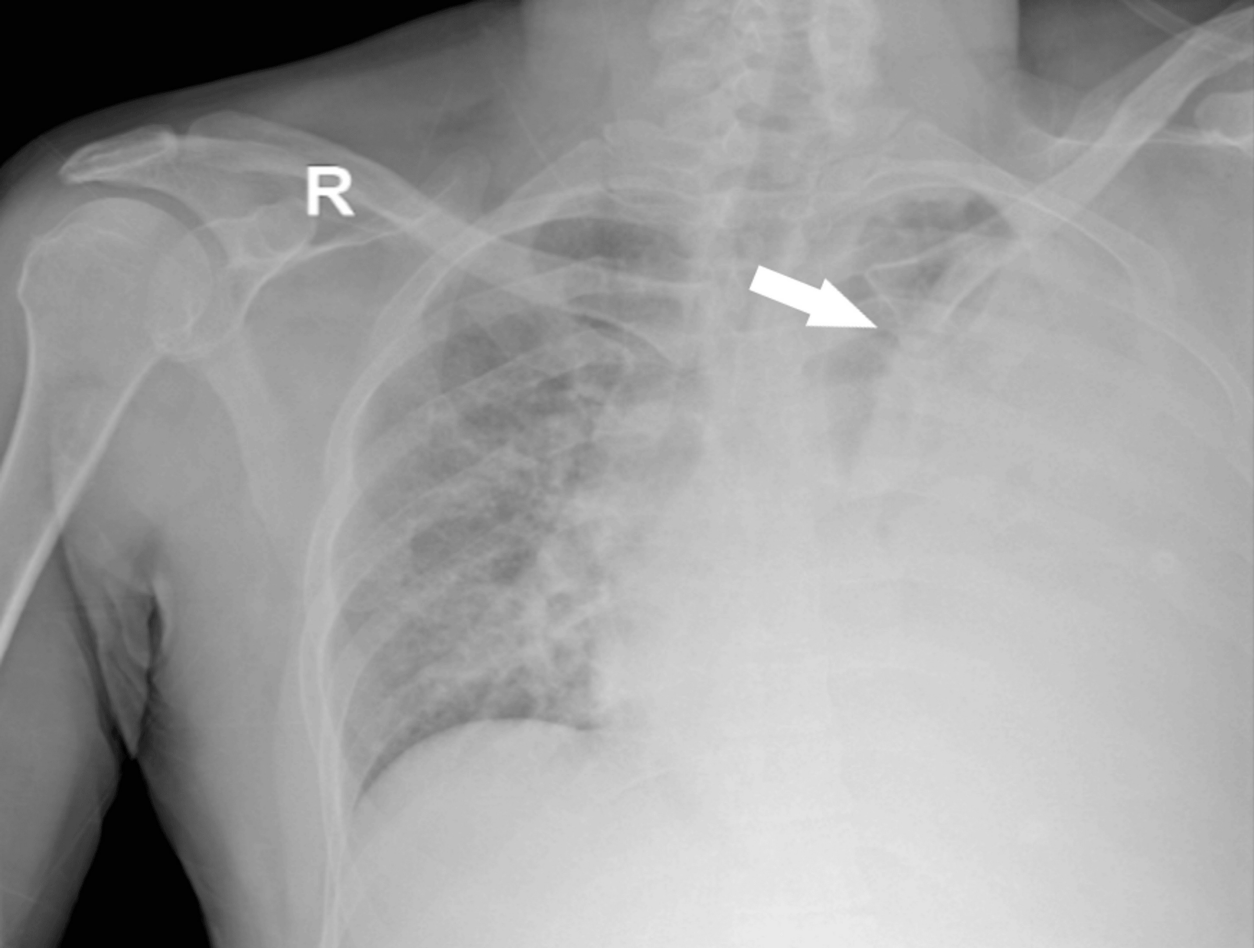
Initial chest X-ray. Chest X-ray showing diffuse opacity throughout the left lung, with sparing of a small apical segment.
